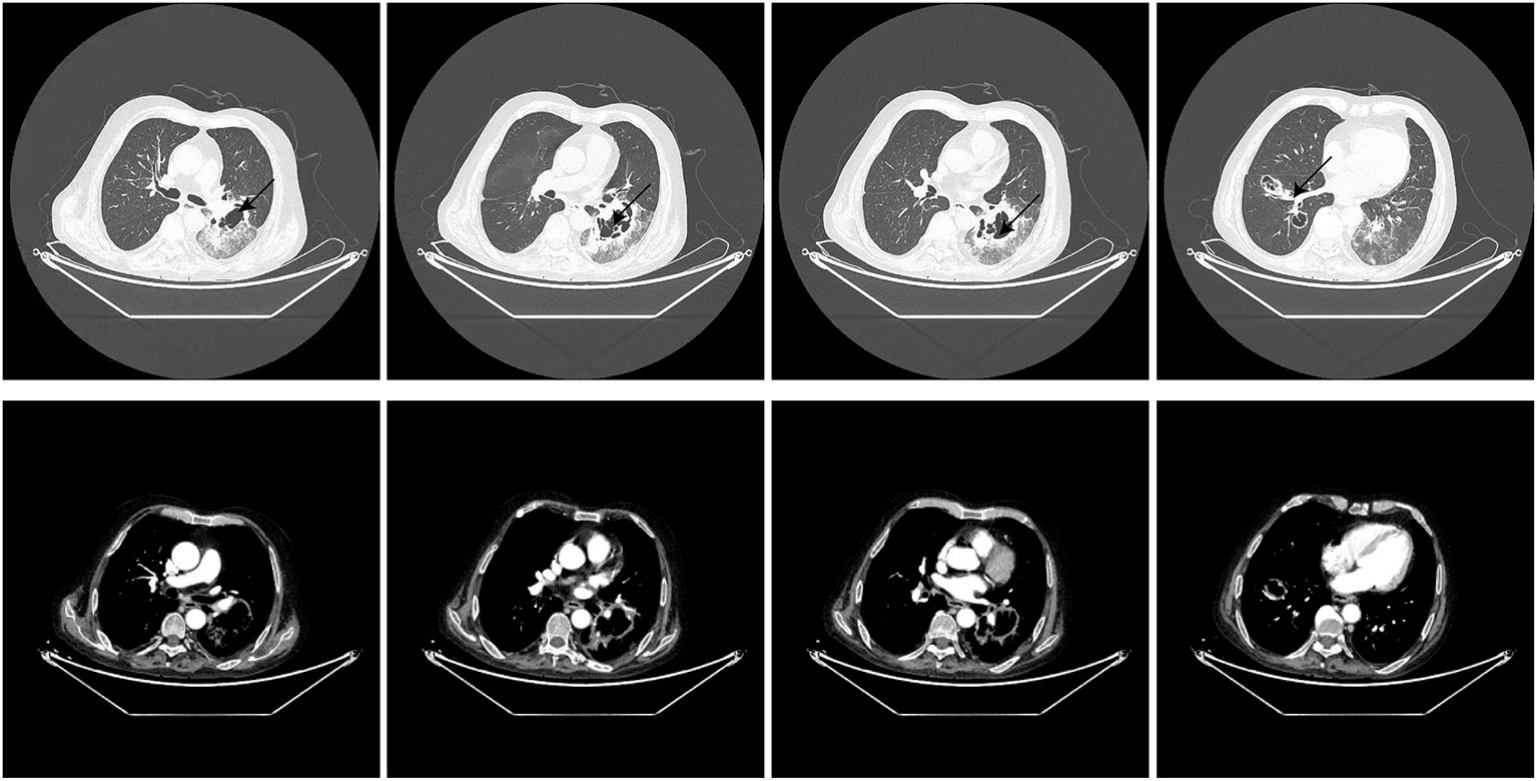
Chest CT upon admission to the hospital.
